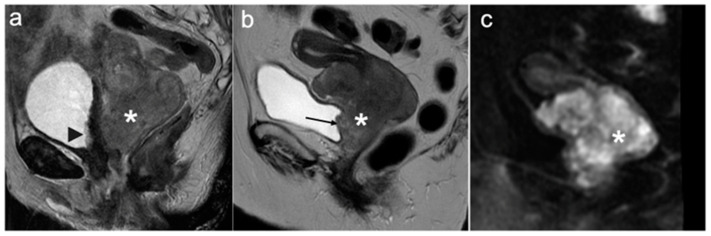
Sagittal T2WI (a) showing a heterogeneous intermediate signal intensity bulky cervical tumour (*). Irregular high T2 signal intensity seen along the posterior bladder wall consistent with bullous oedema (arrowhead). No tumour signal intensity is seen protruding into the bladder. Sagittal T2WI (b) with corresponding DWI (c) of a different patient demonstrating intermediate signal intensity bulky cervical tumour with corresponding diffusion restriction (*). Intermediate tumour signal intensity is seen to disrupt the low signal intensity of the posterior bladder wall and protrudes through the posterior bladder mucosa into the lumen (arrow) consistent with bladder invasion. FIGO stage IVA.
